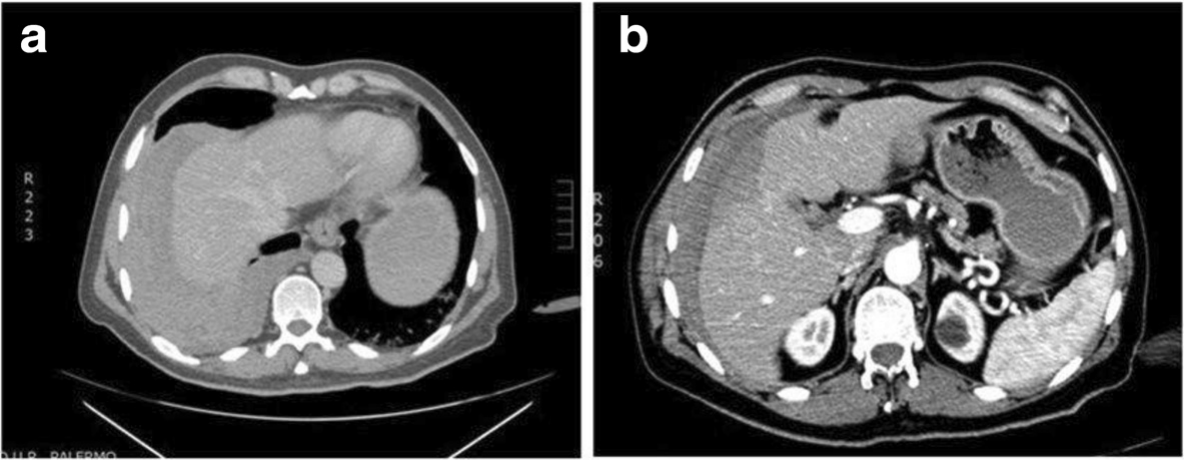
Computed tomography results of the patient. a) presence of a right hemothorax without pulmonary lesions; b) discrete hemoperitoneum by an active bleeding parenchymal liver laceration and “free air” in the abdomen.
